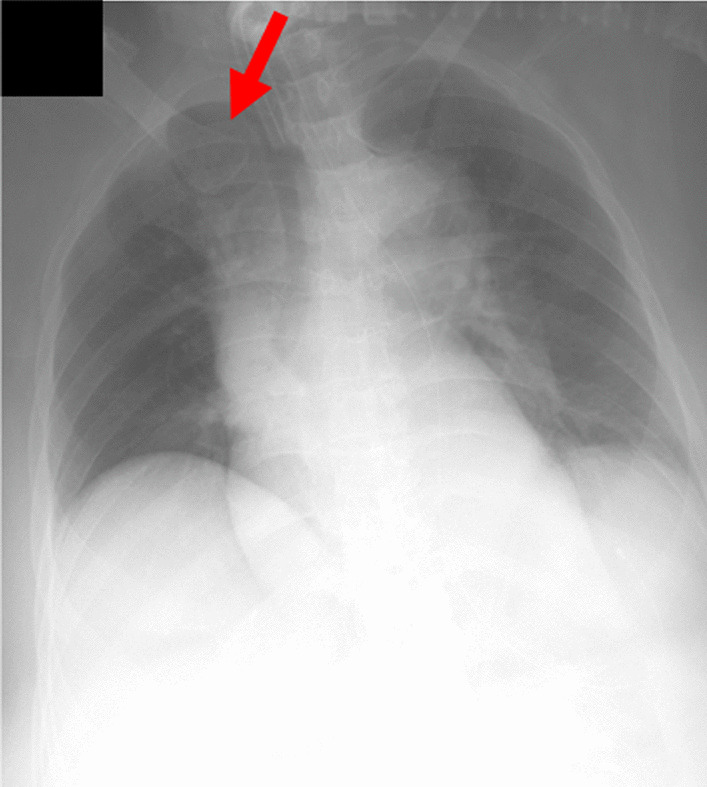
Chest radiograph after 6 months of pulmonary rehabilitation. Red arrow indicates amelioration of atelectasis
